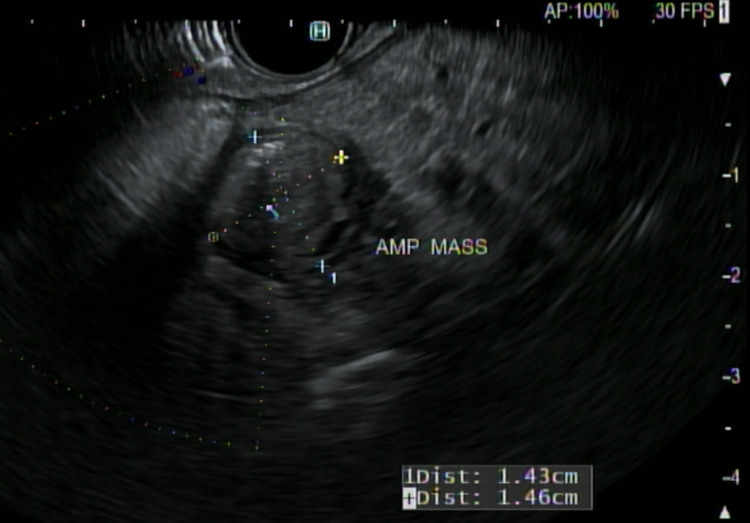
Endoscopic ultrasound shows single intramural mass in the area of papilla.
